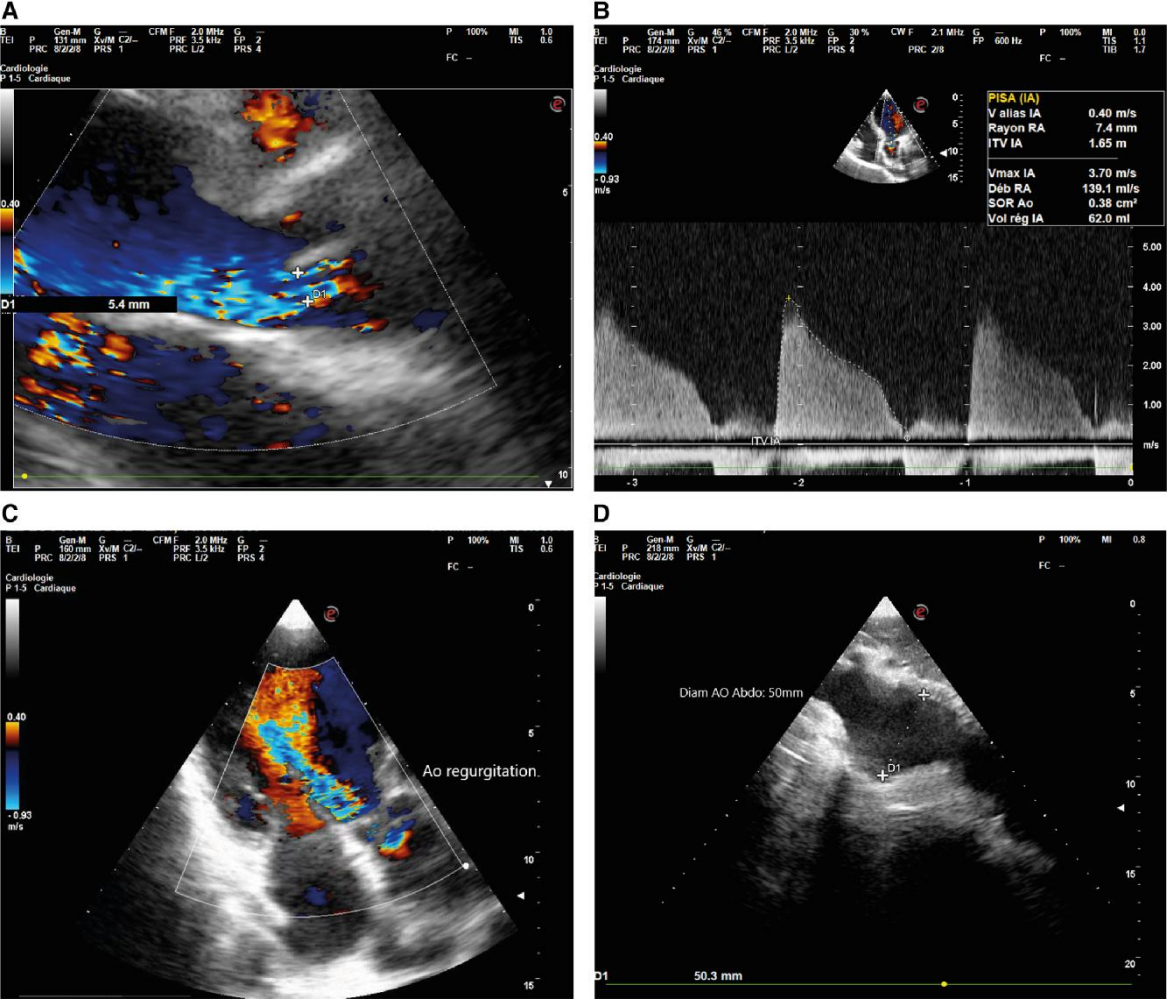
(*A*–*C*) Ultrasound image showing significant aortic insufficiency on a supple tricuspid valve, suggesting inflammatory damage to the valve. (*D*) Partially thrombosed dilation of the abdominal aorta.
